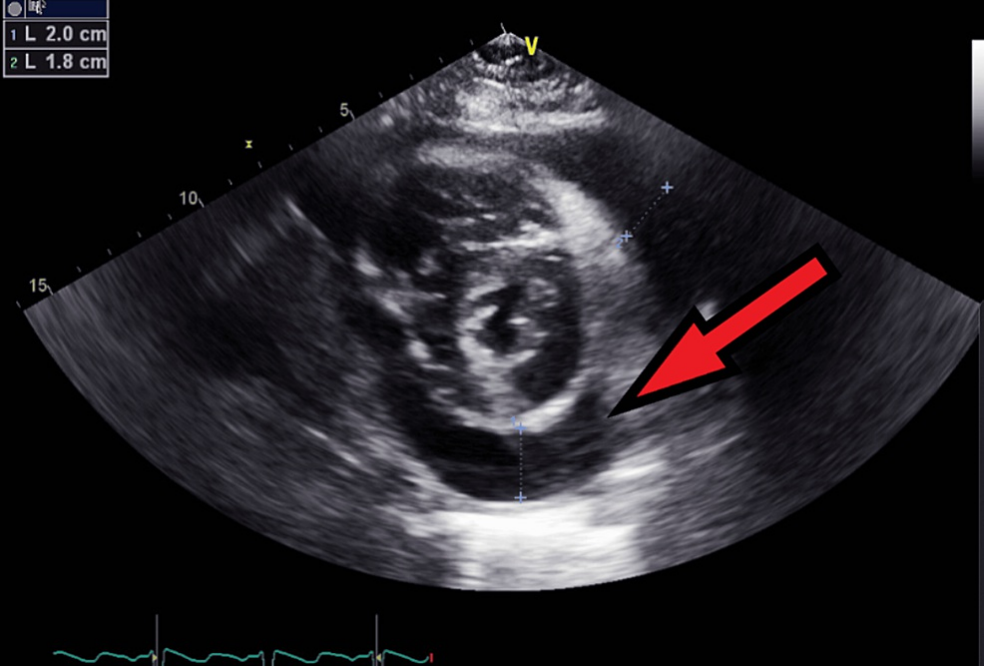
Transthoracic echocardiography on the next day after admission - image 3 Echocardiography on the next day; parasternal short axis view shows increased pericardial fluid collection (red arrow), measuring about 2 cm, indicative of large pericardial effusion
